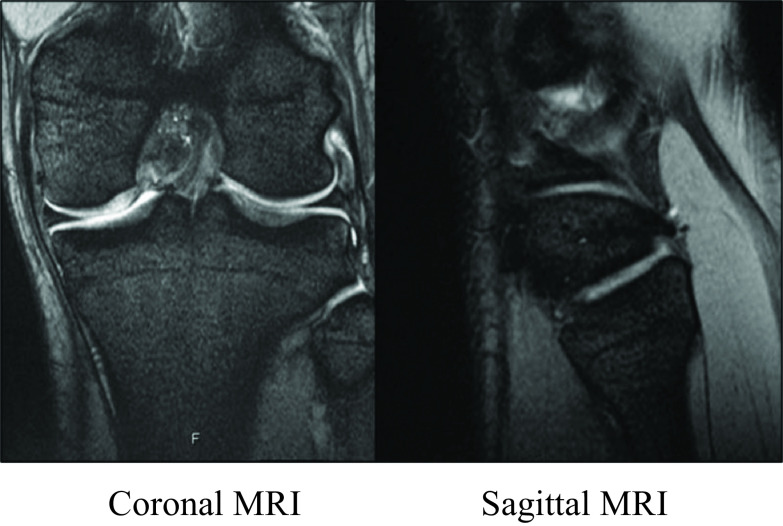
T2-weighted MRI findings. No obvious lateral meniscus injury and cartilage injury was shown on T2-weighted MRI.
